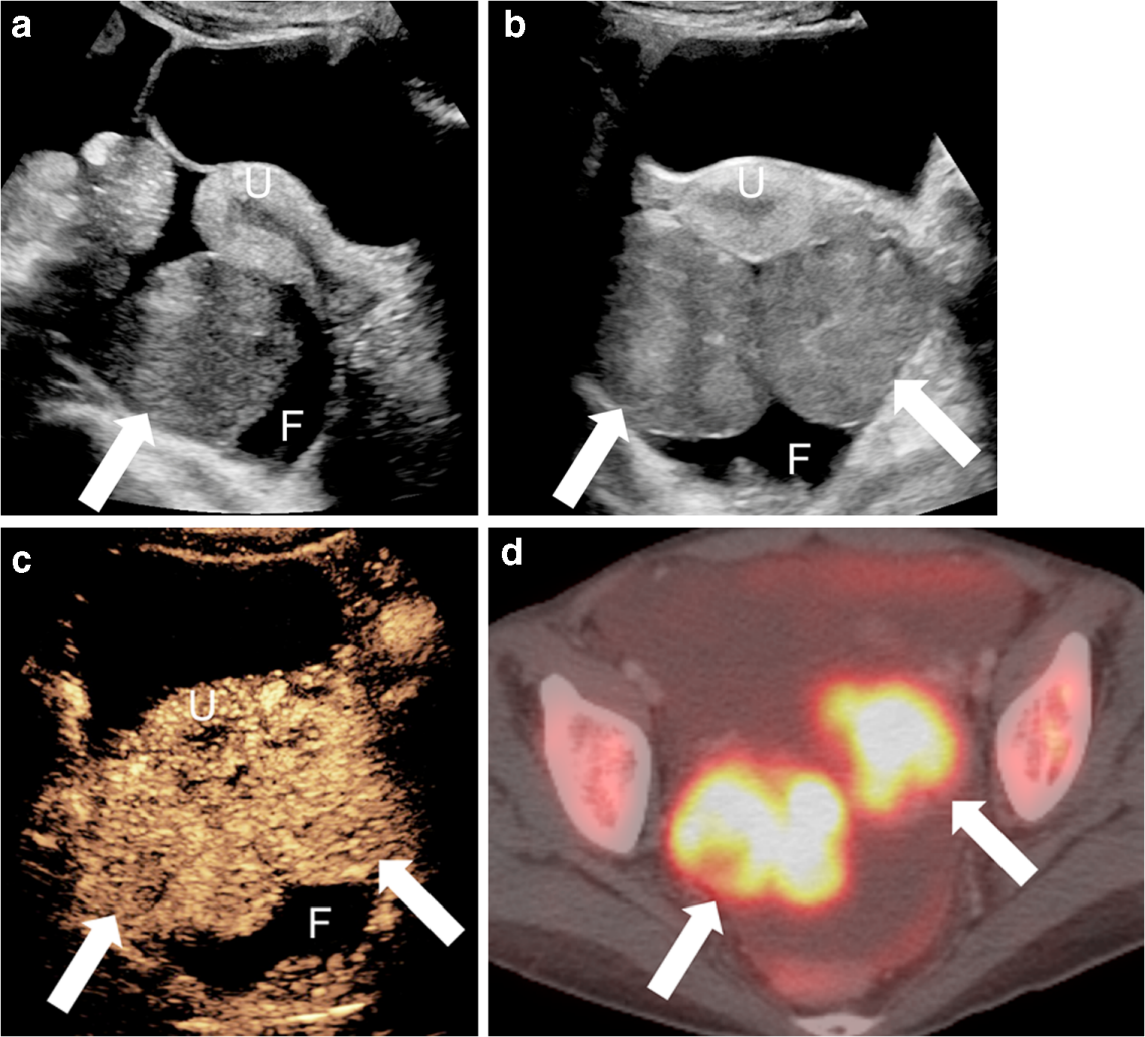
Imaging in an 18-year-old woman with cystic fibrosis status post lung transplant 4 years prior; she had a mediastinal mass concerning for post-transplant lymphoproliferative disease (PTLD). a, b Sagittal (a) and transverse (b) trans-abdominal gray-scale US demonstrates soft-tissue masses (*arrows*) posterior to the uterus (*U*) and small volume of free fluid (*F*) within the pelvis. c Transverse contrast-enhanced ultrasound (CEUS) of the pelvis in contrast-only mode at 2 min 30 s after contrast administration demonstrates heterogeneous enhancement of both ovarian masses (*arrows*), with no normal ovarian follicles visualized. d Axial positron emission tomography (PET)/CT demonstrates marked [F-18]2-fluoro-2-deoxyglucose (FDG) avidity of both ovarian masses (*arrows*), compatible with PTLD
